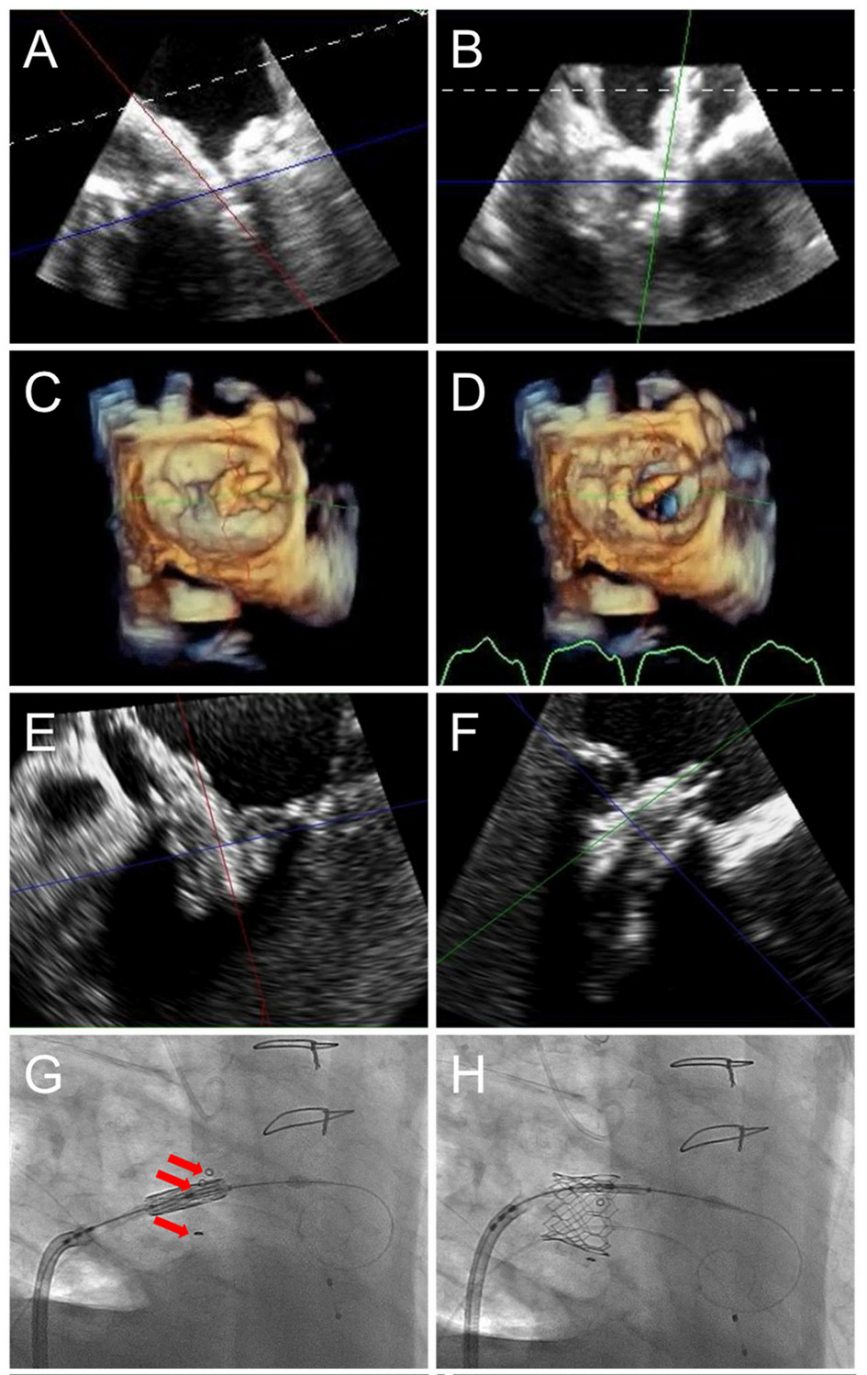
TMVR device positioning. As the orientation of the device may differ from that of the ultrasound plane, live TEE 3D MPR is helpful to properly assess positioning in regards to a calcified mitral annulus (ViMAC) or prior surgical ring (ViR) (A,B). Valve deployment can also be visualized in an en-face surgeon's view (C,D, see Supplementary Video 2). In the case of a valve in valve implantation, a 26 mm Sapien S3 valve is seen being positioned inside a 29 mm bioprosthetic surgical valve in 3D MPR (E,F), confirming alignment of the ventricular edge of the THV valve frame with the ventricular edge of the existing bioprosthesis which can be identified in fluoroscopy (G, red arrows). During deployment (H), the THV shortens predominantly from the inflow (atrial) side, leading to its final position. Supplementary Video 2 demonstrates THV deployment in live 3D MPR.
